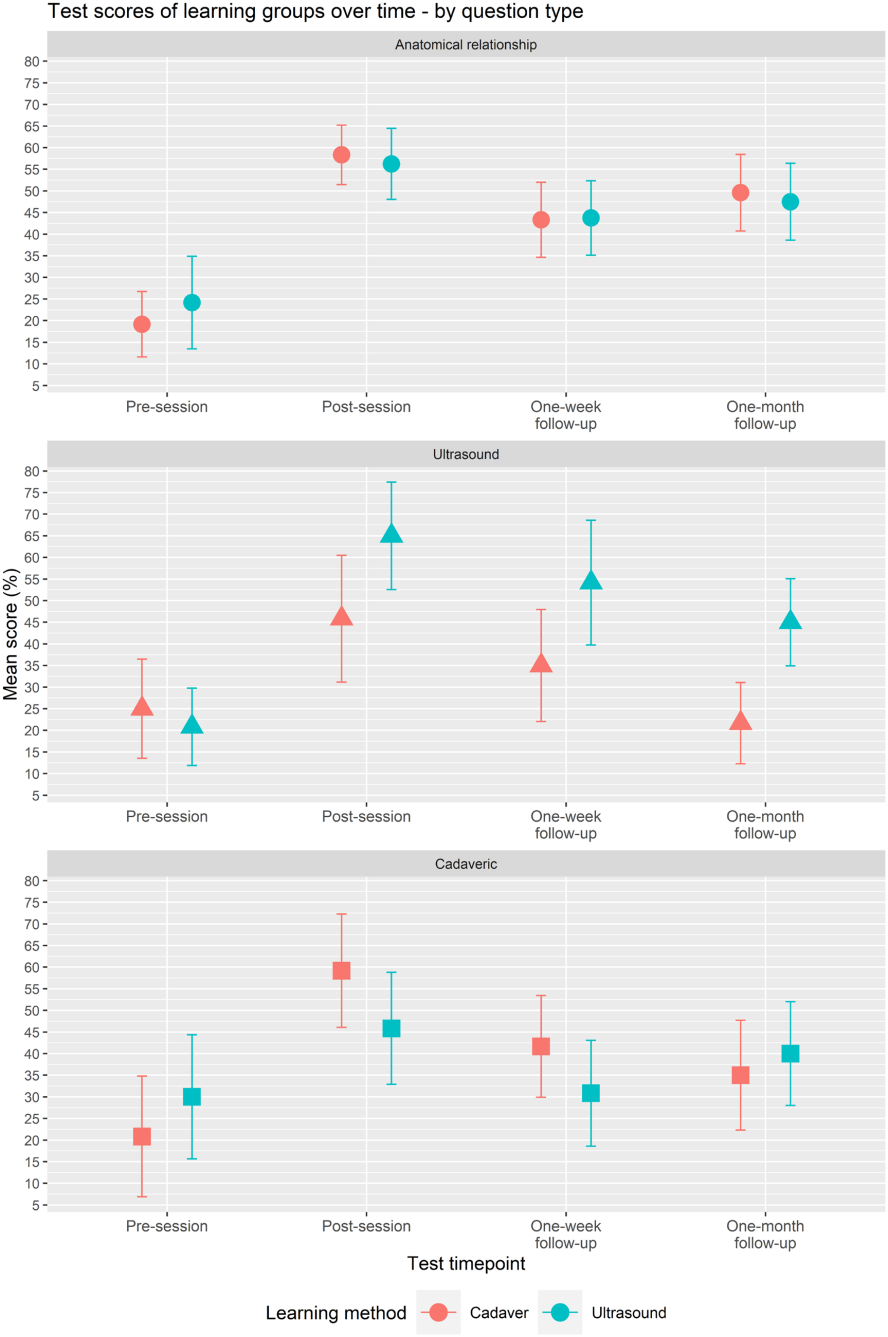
Mean knowledge test scores for specific question types (anatomical relationship, ultrasound, and cadaveric) at each time point throughout the study are shown in red for the cadaver‐based learning group, and blue for the ultrasound‐based learning group. Whiskers represent the 95% confidence intervals for each of the scores.
